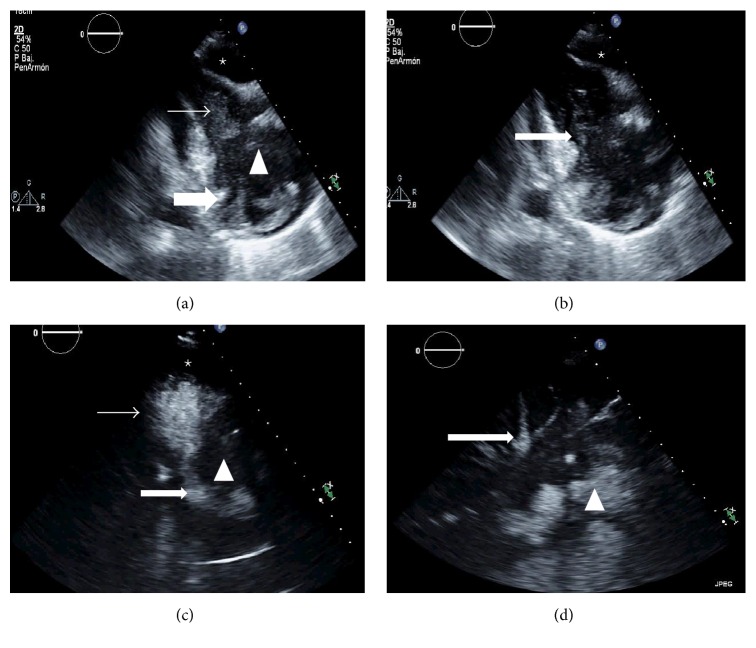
Transthoracic echocardiography with SonoVue® contrast enhancement: (a) shows a homogeneous mass (arrowhead) in the right atrium (RA, thick arrow) which hindered blood flow to the right ventricle (RV, thin arrow), containing contrast medium. Pericardial effusion (star). (b) Same plane image showing stenosis at the tricuspid valve (arrow), with some contrast passing to the RV, as well as tricuspid stenosis. Pericardial effusion (star). (d) Echocardiogram showing contrast in most of the RV (thin arrow) and RA (thick arrow). The arrowhead refers to the homogeneous atrial mass. Pericardial effusion (star). (e) Subcostal plane, showing the contrast (arrowhead) and reflux into the suprahepatic veins (arrow).
